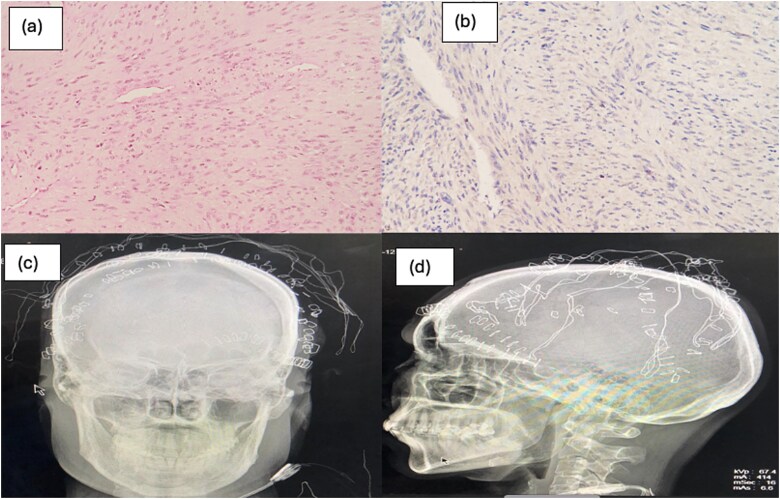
(a, b) Tumor cells arranged in fascicles and herring bone pattern and slit like and staghorn blood vessels; (c, d) post op skull X-ray anteroposterior view and lateral view, respectively, showing marking of deep posterior margin of excised tumor.
